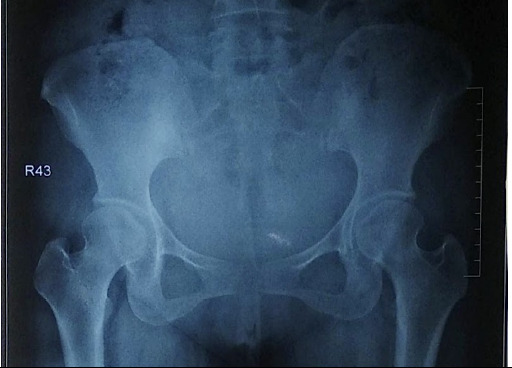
Anteroposterior radiograph of the pelvis at discharge.
